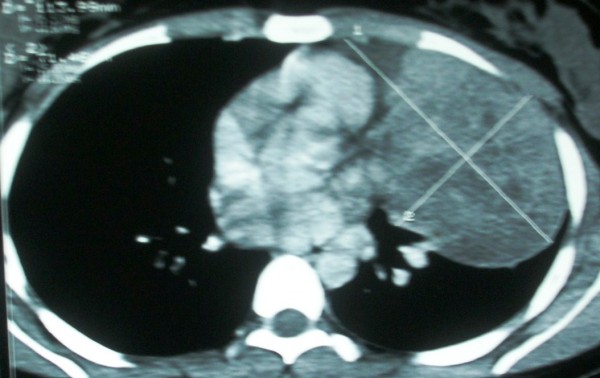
CT chest showing tumor in left upper lobe.
